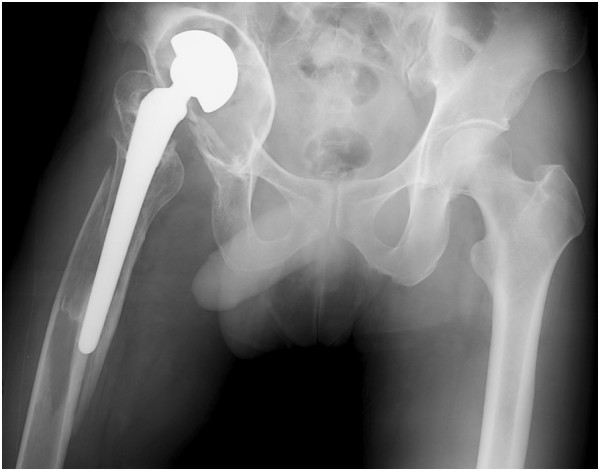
X-ray showing a peri-prosthetic fracture of the femur.
